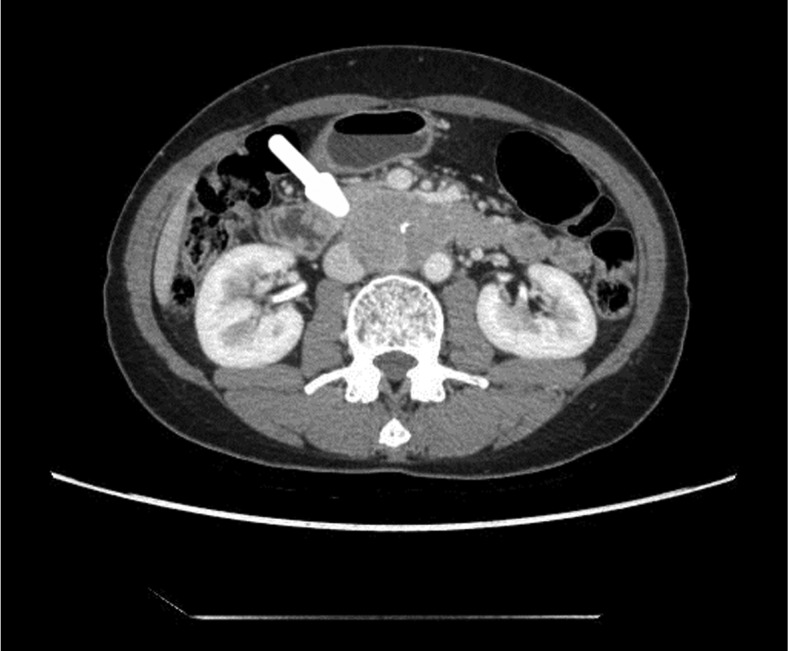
Abdominal computed tomography scan revealing a 5×6cm tumor. Arrow indicates the tumor.
